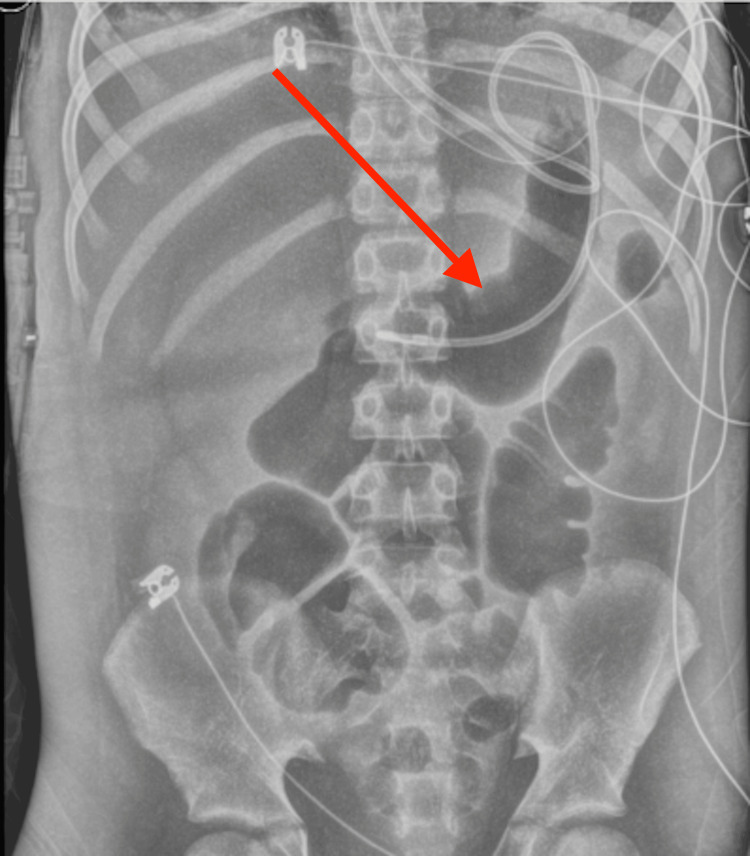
Portable supine abdominal radiograph suggesting ileus This image shows multiple dilated gas-filled bowel loops suggestive of ileus in the appropriate clinical context. An enteric tube projects over the stomach.
